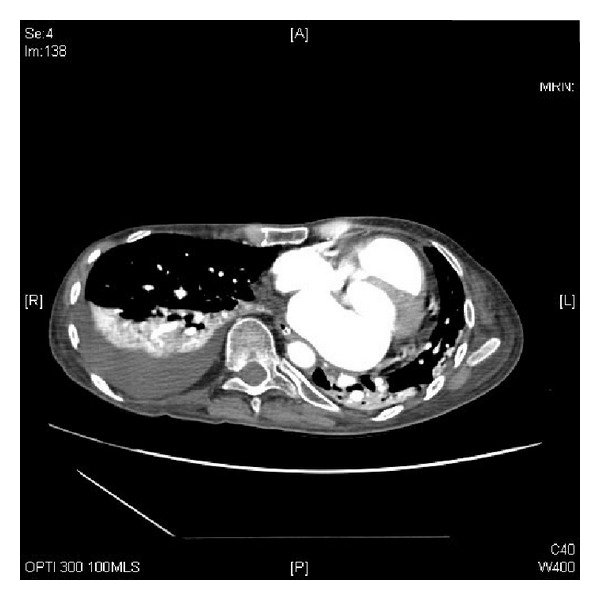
CT of chest, performed on 05 August 2009, revealed atelectasis and consolidation posteriorly in the right upper lobe and in both lower lobes. There was a moderately large right-sided pleural effusion.
